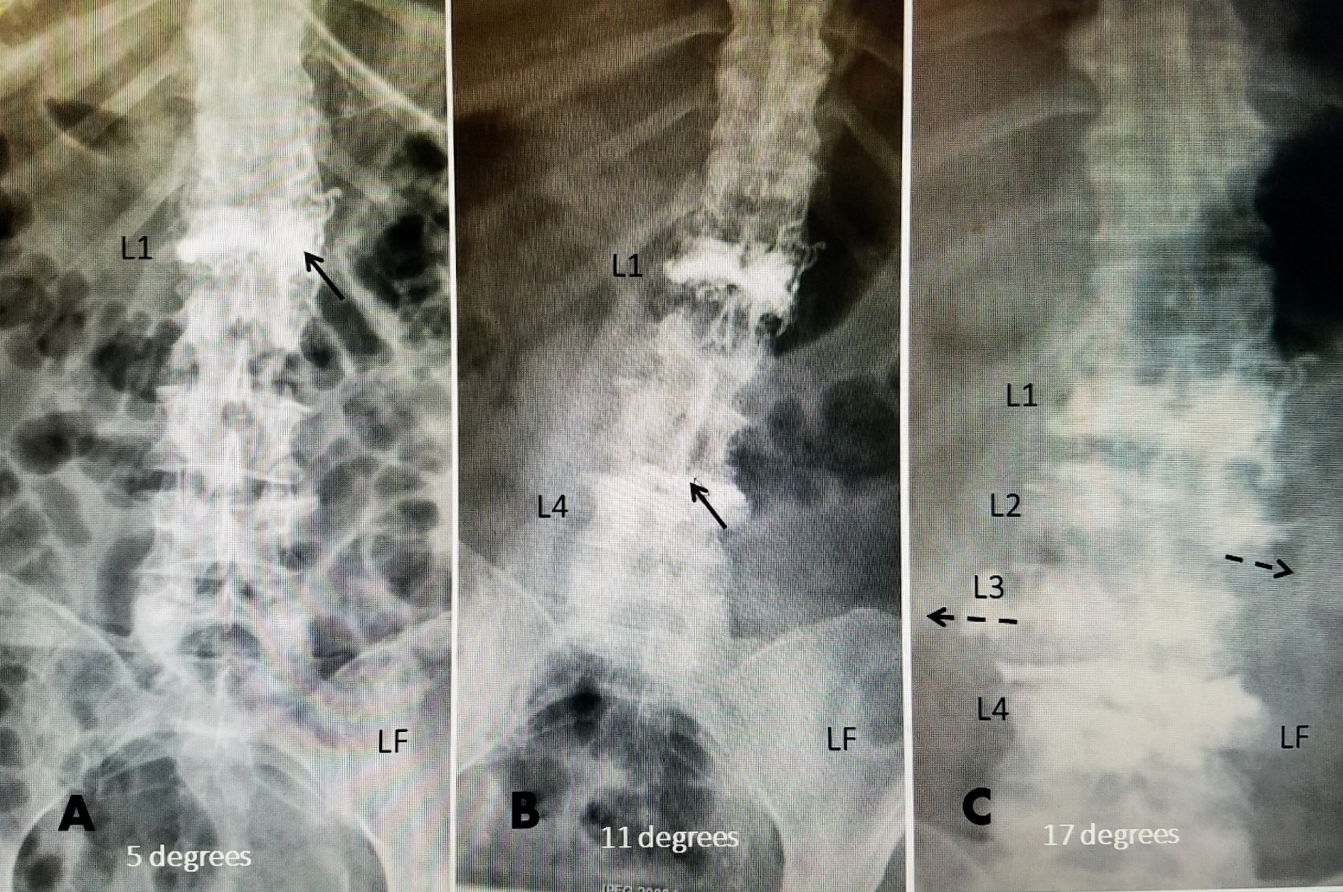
Progressive degenerative scoliotic deformity from multiple lumbar compression fractures The patient is a 63-year-old female on chronic steroids for Sjogren's syndrome. She was taking alendronate 70 mg before the initial fracture. Her bone mineral density on medical therapy was -2.9. A: Initial radiograph showing L1 vertebral kyphoplasty (black arrow) and mild 3^o ^angulation to the left at L3 and slightly to the right at L1-2. B: Nine months later, after a pain-free interval, she had another minor fall. She developed a second fracture at L4, treated with vertebral augmentation with polymethyl-methacrylate (PMMA) (black arrow). There now is an 11^o^ angulation. She was placed on a teriparatide 20 ugm daily injection. She had residual low-grade back pain after the second kyphoplasty but was fully active. C: Eighteen months later, the patient started to complain of more constant back pain without a specific fall. Studies showed a worsening of the lateral scoliosis with the coronal angulation increased to 17^o^. A bone scan showed new fractures at L2 and L3. She was treated with a two-level vertebroplasty and vertebral augmentation with Cortoss (Stryker Corporation, Kalamazoo, Michigan, USA), had a residual dull lumbar pain, and was advised to use a lumbar sacral orthosis (LSO).
